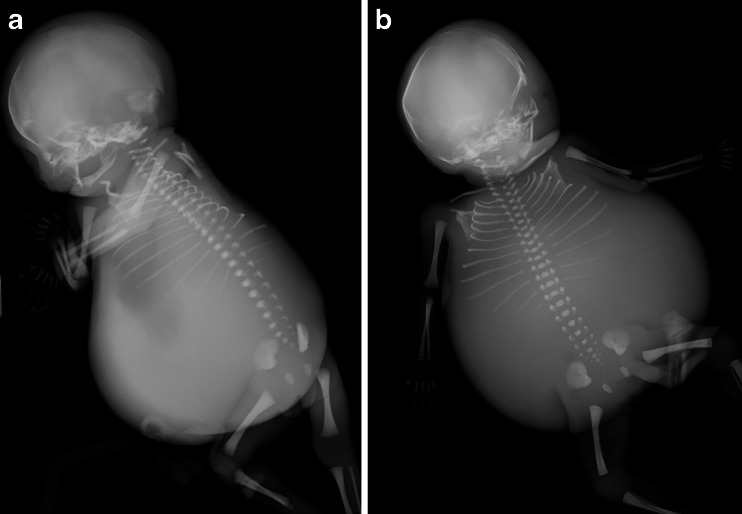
Case 1. Postmortem radiography in a 24-week male foetus with congenital high airway obstruction syndrome. a Lateral and (b) anteroposterior postmortem skeletal radiographs show gross soft-tissue swelling of the thorax and abdomen, with splaying of the ribcage. No limb anomalies were identified in this foetus
